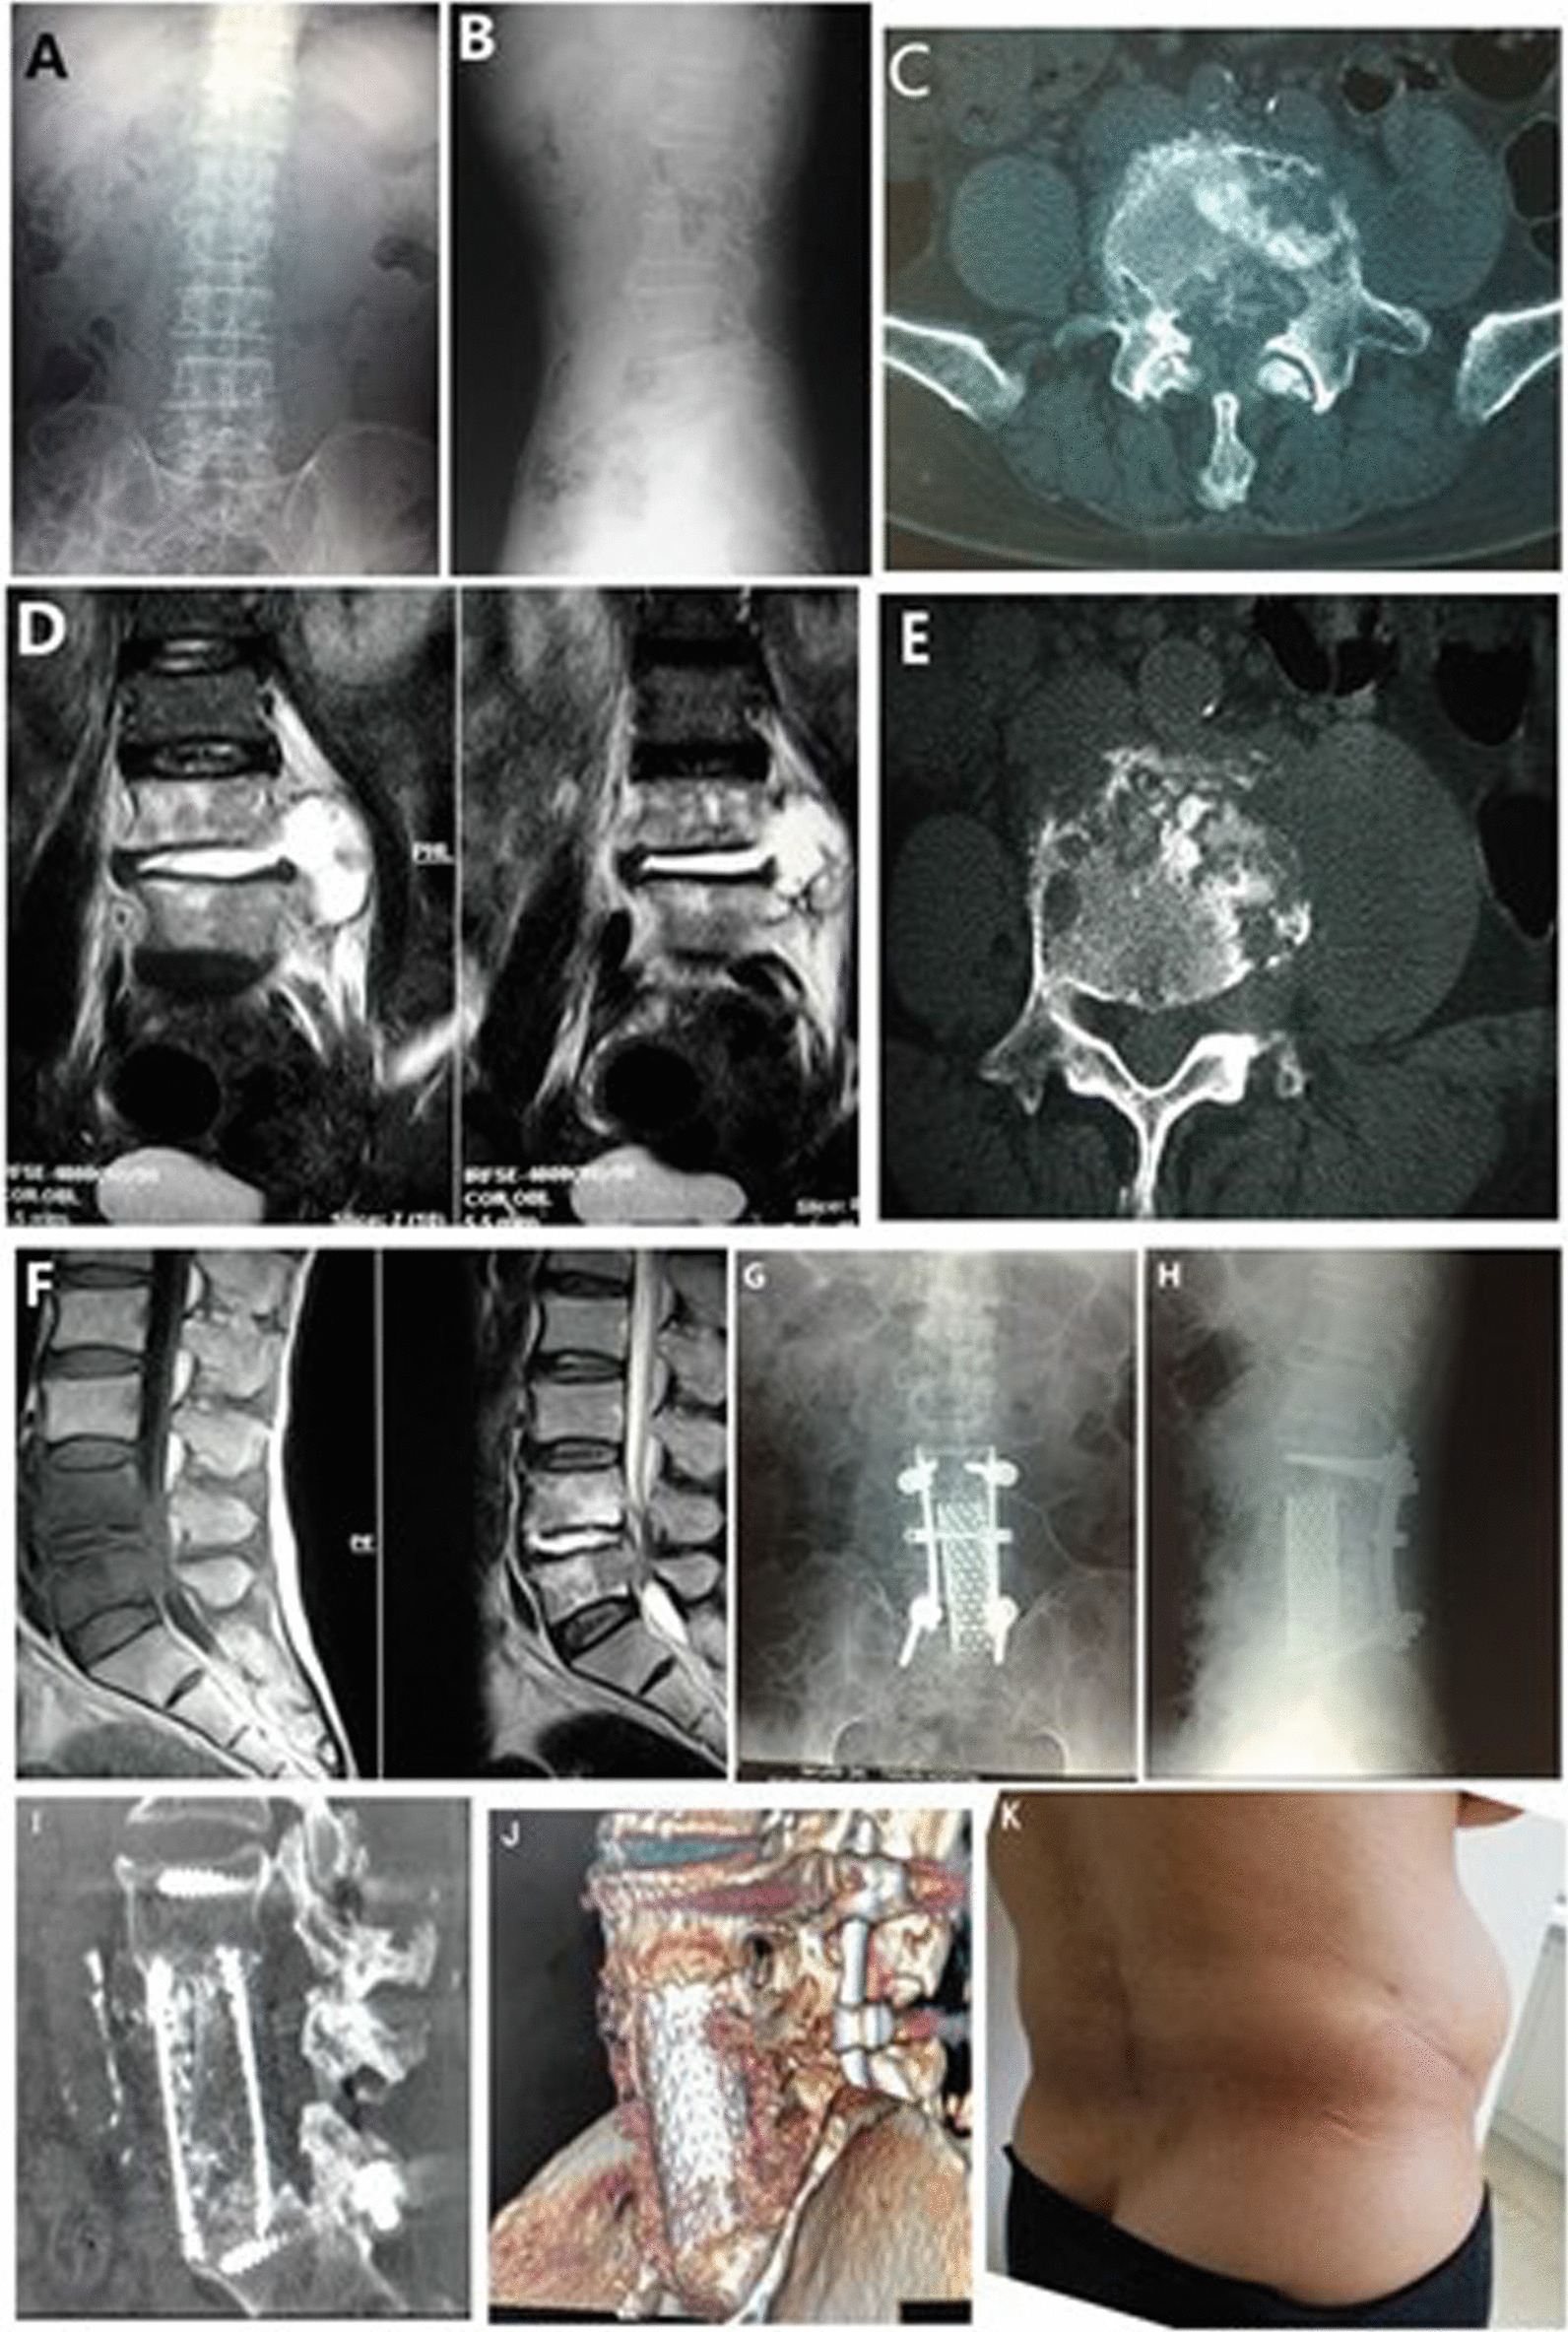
A 65-year-old man L4-5 TB. Preoperative, posteroanterior A and lateral B plain radiographs. Cross-sectional CT scan C, E and MRI D, F views showed bony destruction form the L4–L5 vertebrae, with prevertebral and paravertebral abscessed compressing the neural elements. Postoperative radiographs include anteroposterior G and lateral (H). The last folloe-up 3D CT showed good fusion (I, J). Photo of the patient’s incision at the last review (K)
